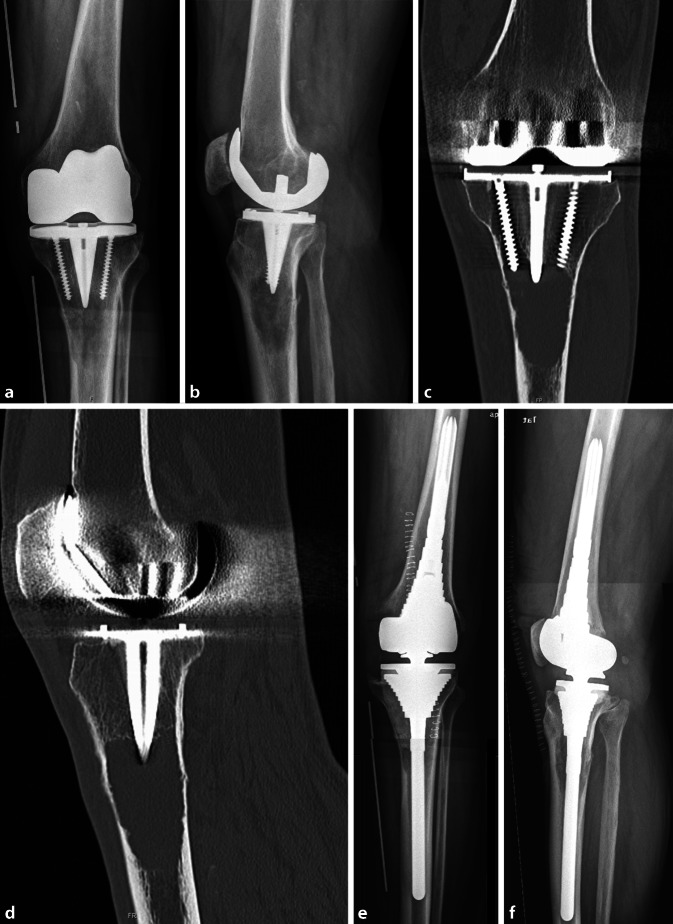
X‑ray radiographs (a anteroposterior, b lateral view) of aseptic loosening of primary total knee components with major osteolytic changes in the proximal tibial diaphysis due to wear of the inlay (particle disease) (courtesy of the Department of Diagnostic and Interventional Radiology, University Hospital of Leipzig, all rights reserved). c, d  Prior to surgery (c anteroposterior, d lateral view) a CT scan was performed to confirm the diagnosis and rule out malignant transformation (courtesy of the Department of Diagnostic and Interventional Radiology, University Hospital of Leipzig, all rights reserved). e, f The same patient (e anteroposterior, f lateral view) after implantation of a rotating hinge knee revision system with metaphyseal sleeves (courtesy of the Department of Diagnostic and Interventional Radiology, University Hospital of Leipzig, all rights reserved)
